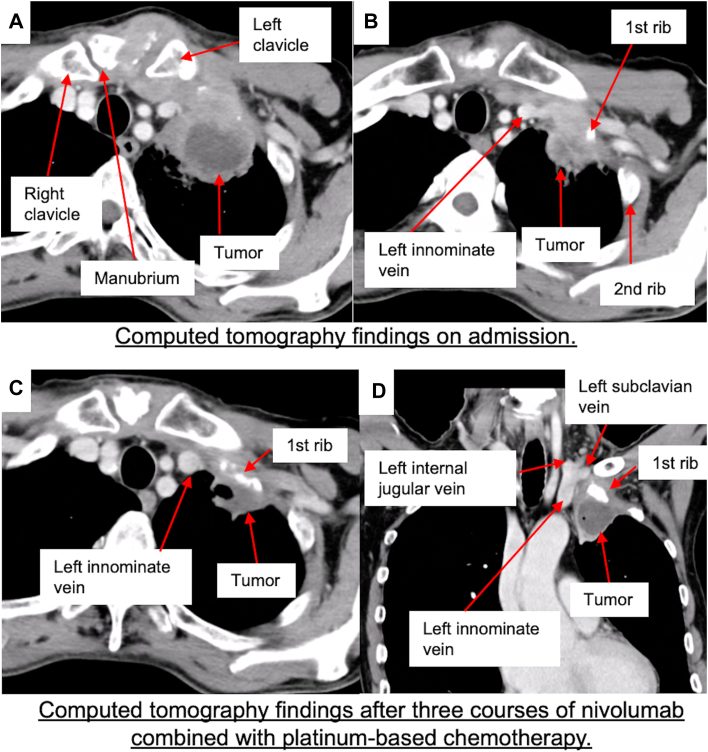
(A, B) Computed tomographic findings on admission. A 107-mm solid mass was observed in the left upper lobe that invaded the manubrium, the left clavicle (A), and the left first and second ribs (B), although no intrathoracic lymph node swelling was detected. (C, D) Computed tomographic findings after immunochemotherapy. The primary tumor showed remarkable shrinkage; however, invasion into the first rib persisted (C). Involvement of the great vessels was unclear (D).
